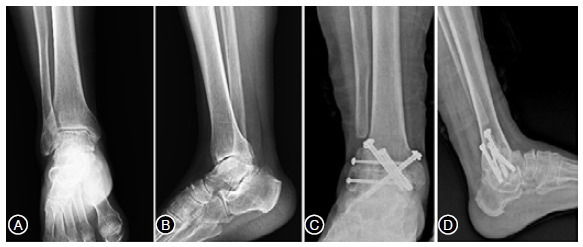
Preoperative weightbearing radiographs of the ankle. Takakura stage 3. (A) anteroposterior X-ray and (B) lateral X-ray. Transfibular approach was performed with three 6.5-mm cannulated screws and two 4.5 cannulated screws with fibular onlay bone graft; (C) anteroposterior view; (D) lateral view showing fusion of the ankle joint at the final follow up (11 months after operation).
